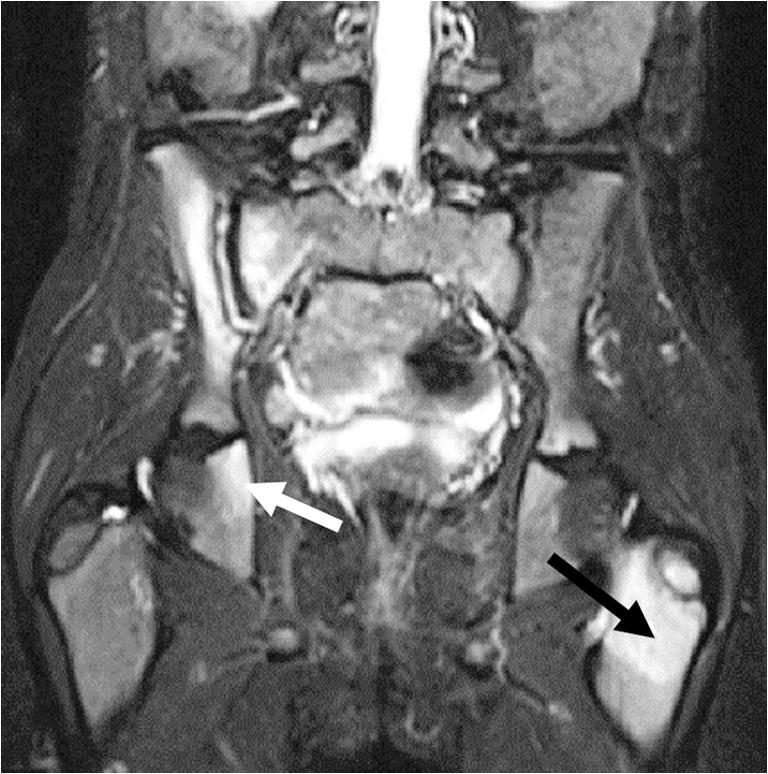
Chronic nonbacterial osteomyelitis in a 7-year-old girl. Coronal T2-W short tau inversion recovery MR image (repetition time/echo time = 5,000/58 ms) shows involvement of the right sacroiliac joint (high signal in the joint space with surrounding bone marrow edema), the right ischial bone (*white arrow*) and the left femoral metaphysis/apophysis (*black arrow*). Note the subtle high signal of the right femoral metaphysis, within normal variation
